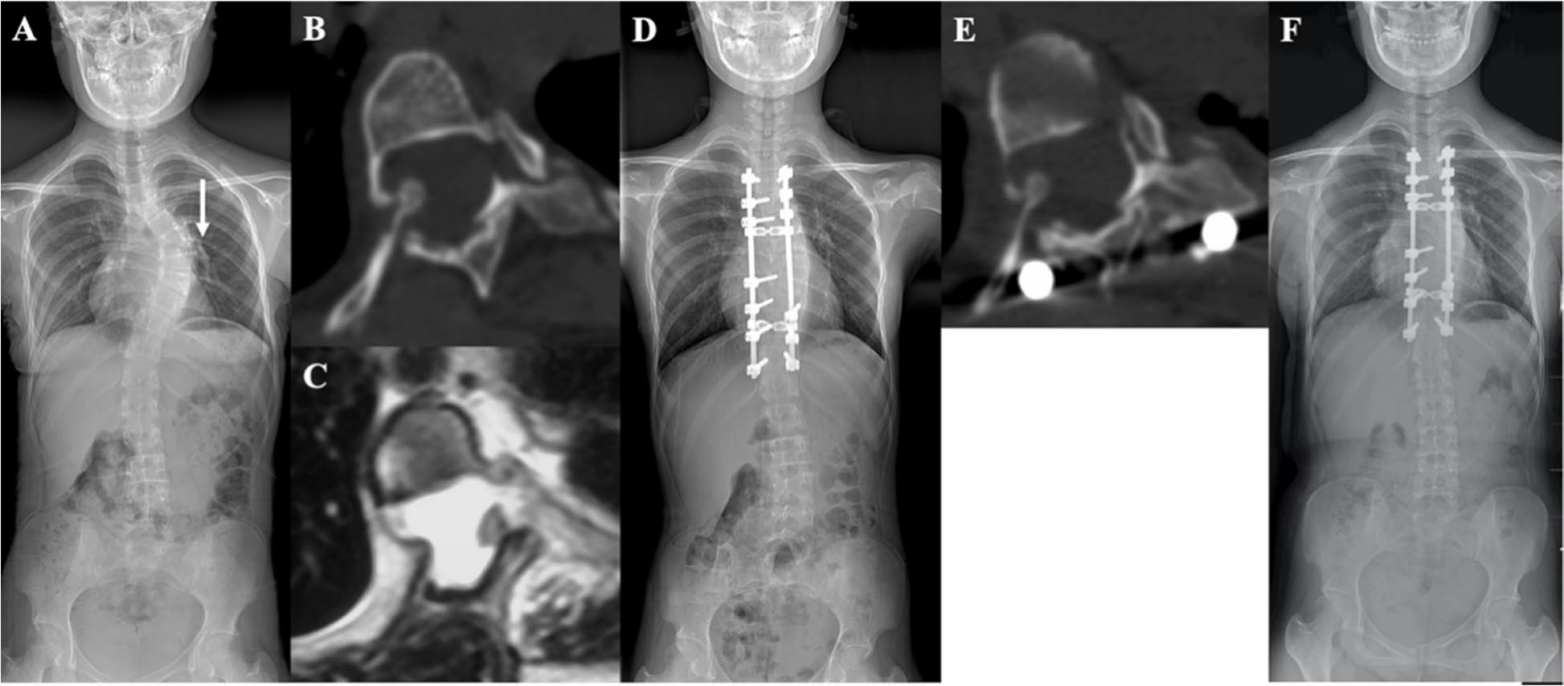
A 14-year-old boy with left thoracic dystrophic scoliosis secondary to NF-1 received surgical treatment in our institution. A The preoperative Cobb angle of thoracic curve was 60° with 8^th^ rib head dislocation (white arrow) and the VT of T8 was 12.2 mm B and C. Preoperative CT and MRI scans revealed the 8^th^ rib head penetrating the foramen and compressing the dural sac but not the spinal cord. The IRL and the VRA were 12.2 mm and 75.3°, respectively. D Posterior-only spinal fusion without rib head excision was performed, and pedicle screw or hook was not placed at the level of dislocated rib head. The Cobb angle was corrected to 31° postoperatively. E A minor degree of rib head withdrawal was confirmed by postoperative CT scans. The IRL, RVA and VT were corrected to 9.9 mm, 69.6° and 12.4 mm postoperatively
